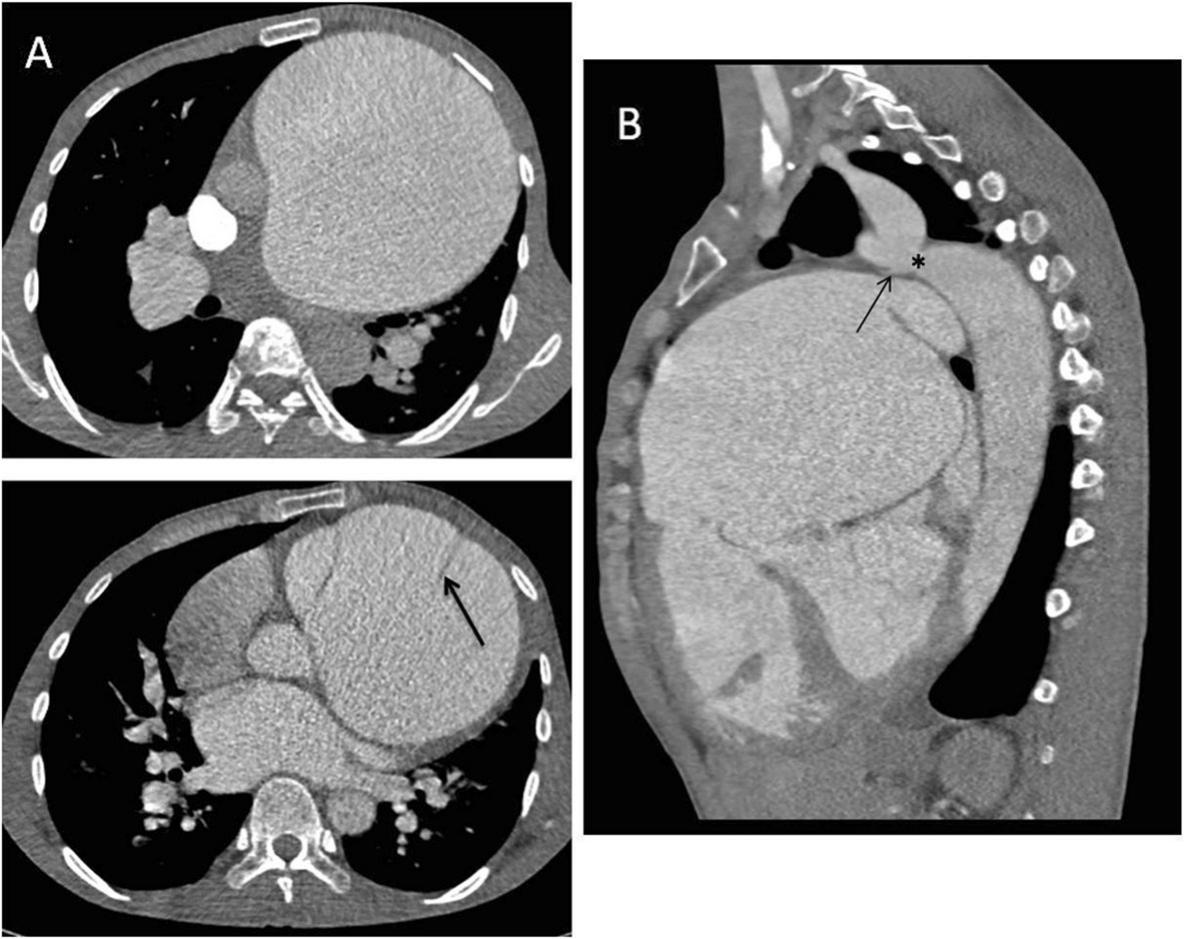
Computed tomography angiography. a Main pulmonary aneurysmal dilation with a diameter of 14 cm. b Aortic coarctation at the aortic isthmus and a small patent ductus arteriosus at the ductus arteriosus. c CTA showing pulmonary artery dissection
